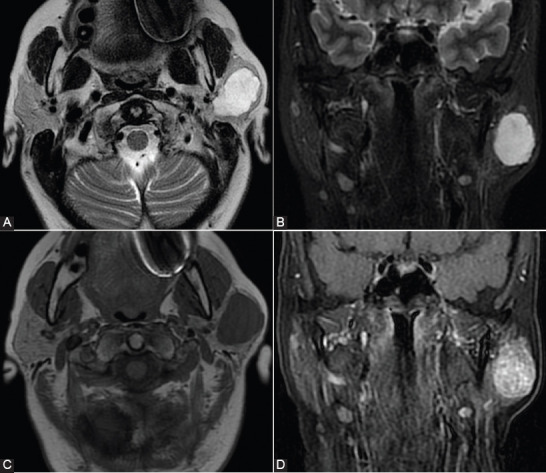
Conventional magnetic resonance imaging appearance in a 60-year-old patient with a left parotid gland pleomorphic adenoma. (A and B) T2-weighted image (WI) sequence and short tau inversion recover (STIR)-WI sequence revealing hyperintense signal. (C and D) T1-WI sequence without contrast and T1 contrast-enhanced sequences showing hypointense signal and homogeneous enhancement.
